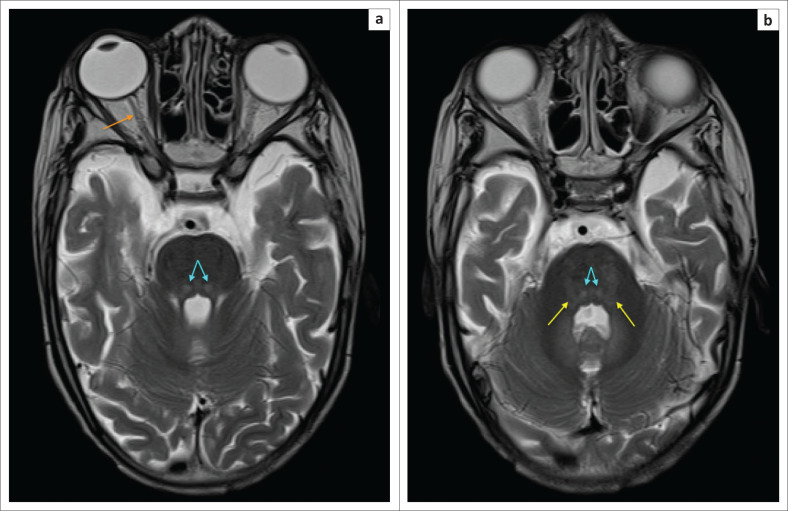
A 7-year-old male child with mixed subtype hypoxic ischemic brain injury. Axial T2-weighted sequence images through the midbrain and pons show dorsal tegmental (yellow arrows) and central tegmental tract (cyan blue arrows) hyperintensity. Note severe cerebral atrophy and optic atrophy (orange arrows).
